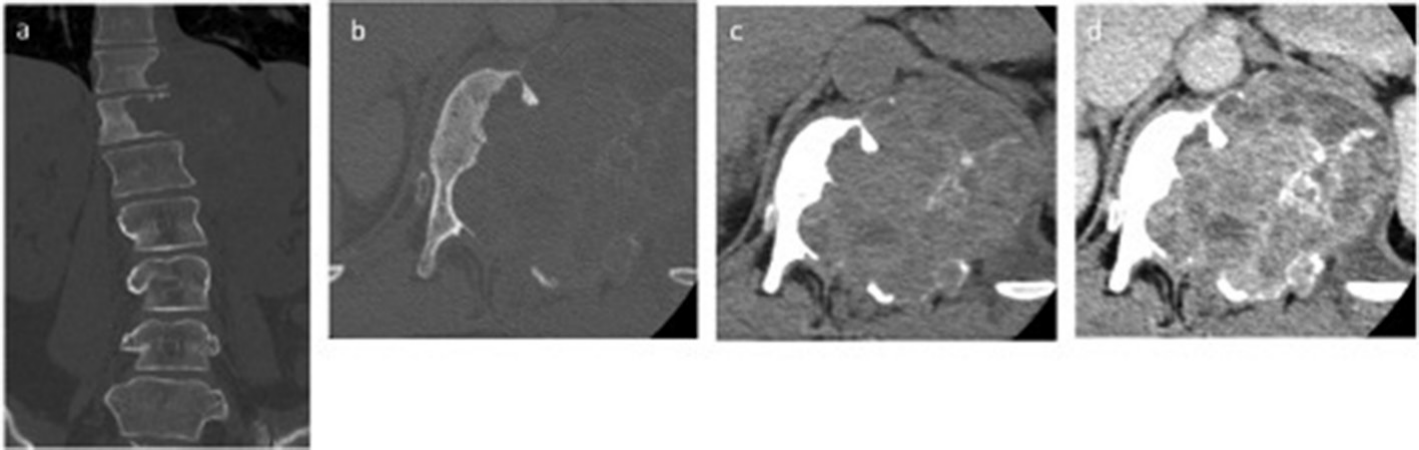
(a–d) (a, b) Non-contrast CT bone windows demonstrates bony destruction at T11 and T12 vertebral level with a large soft tissue mass adjacent to it. There is quite prominent bony destruction but there is well-circumscribed borders on closer inspection. Note fine bony septations in b. (c, d:) Pre- and post-contrast CT studies in soft tissue window demonstrating prominent heterogenous soft tissue enhancement.
